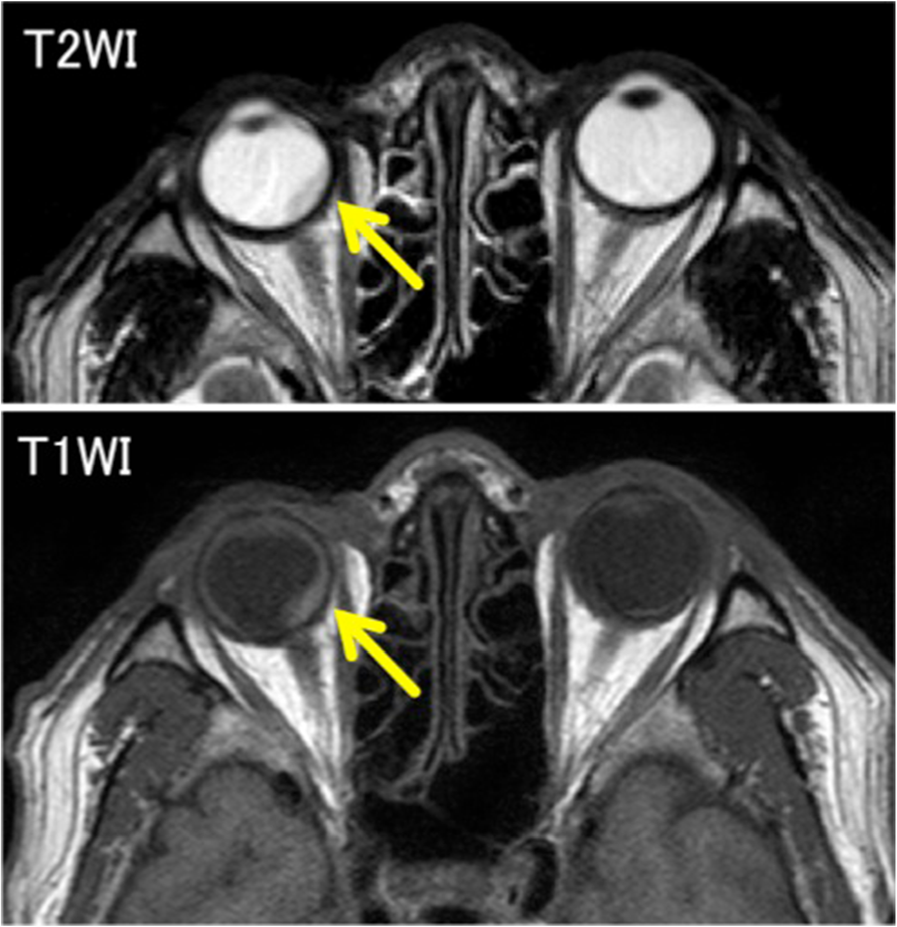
Magnetic resonance imaging (MRI). An elevated choroidal tumor was detected. The T1-weighted image was isointense, and the T2-weighted image was hypointense
